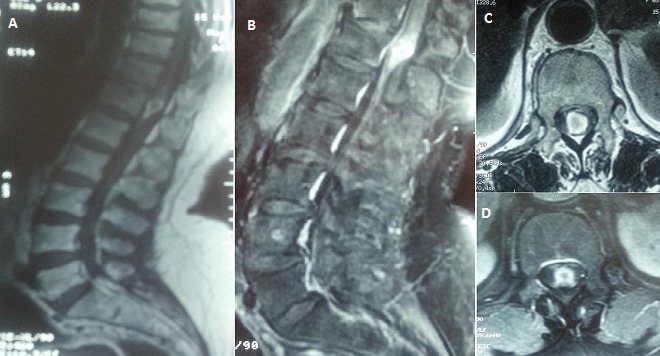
A) sagittal MRI T1 sequence: presence of an epidural haematoma at T12-L1; B) sagittal MRI T2 sequence: epidural haematoma at T12-L1 with spinal cord compression; C) axial MRI T1 sequence: epidural haematoma at T12; D) axial MRI T2 sequence: epidural haematoma at T12, spinal cord compression
